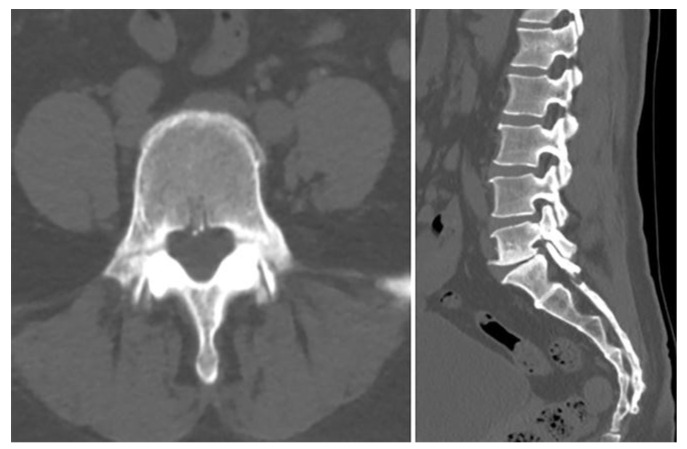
Follow-up CT images showing sclerotic changes around the fracture site with partial bone bridge formation, indicating progressive healing.
